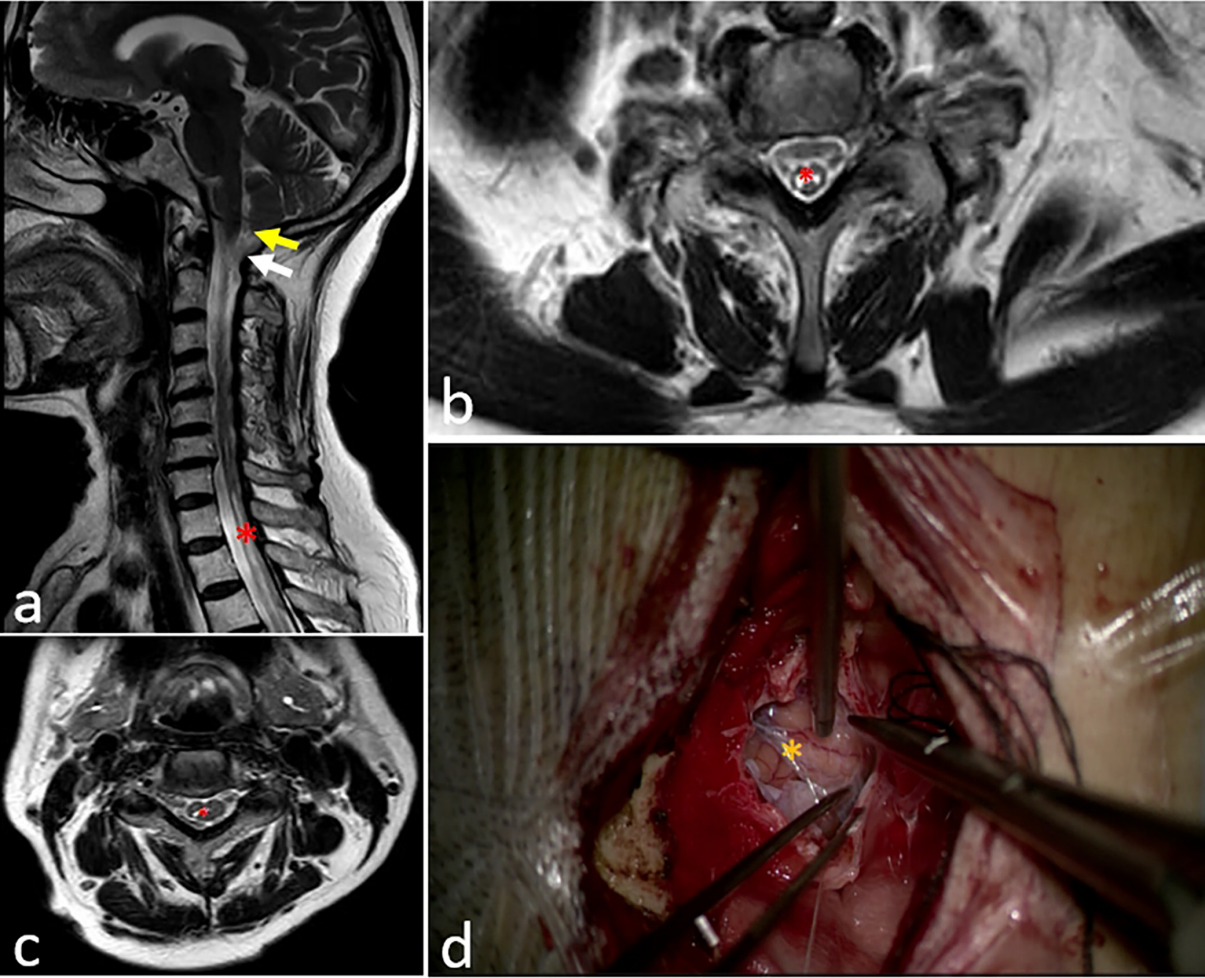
Case presentation of a patient with extensive adhesive arachnoid combined with secondary syringomyelia. A 76‐year‐old female presented with progressive neck pain, weakness, and numbness in her right upper limb for 2 years. The MRI imaging exhibited Chiari malformation type I and extensive adhesive arachnoid with secondary syringomyelia. Foramen magnum decompression was performed. (a) Sagittal and (b, c) axial T2‐weighted spine MRI demonstrating Chiari malformation (yellow arrow) and extensive adhesive arachnoid (white arrow) and syringomyelia (red asterisk); (d) intraoperative view to the sites of adhesive arachnoid (orange asterisk).
